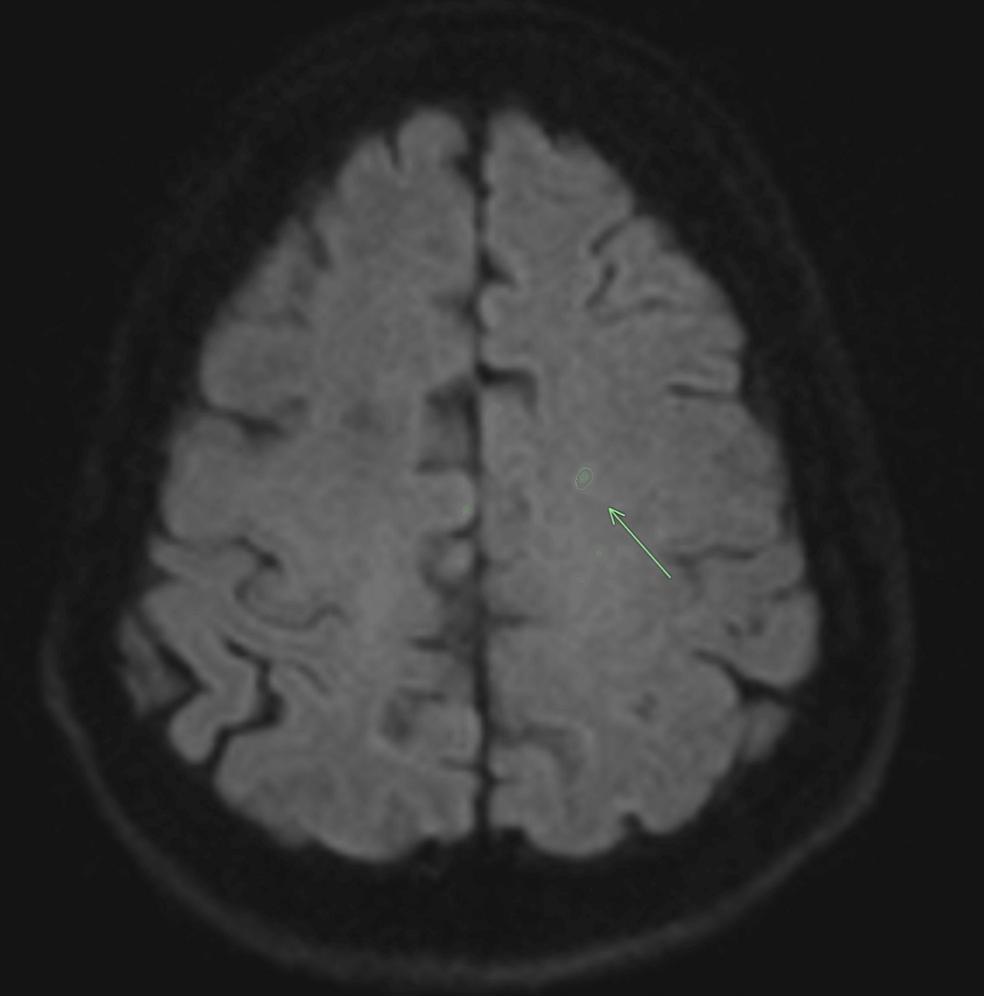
MRI brain without contrast showing a new lacunar infarct in the left frontal lobe.
